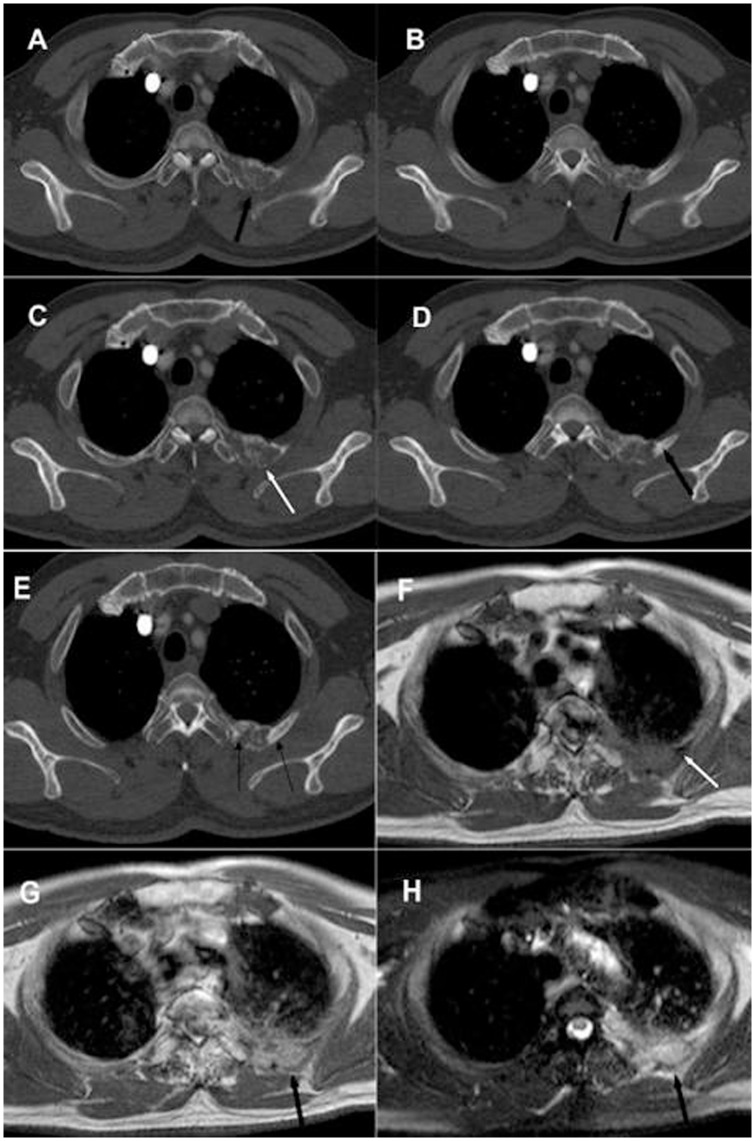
The imaging manifestations of rib metastases in lung cancer, comparing 5 mm and 1 mm slice thickness on CT, and MRI. A, B. Two consecutive 5 mm levels of CT images showing a rib metastasis (black arrow); CT depicted predominantly osteolytic and expansive changes, osteoblastic changes were not obvious. C, D, E. Thickness of 1 mm interception of three discontinuous CT dimensions showing typical mixed changes, osteoblastic changes (black arrow), and osteolytic changes (white arrow). F. T1WI shows an area of isometric and low-mixed signal intensity; the area that showed osteoblastic changes on CT reveals low signal intensity on T1WI (white arrow). G. The lesion enhances heterogeneously on Gd-DTPA enhanced T1WI imaging (black arrow). H. T2WI (FS) shows high, slightly high, isometric and low-mixed signal intensity (black arrow).
